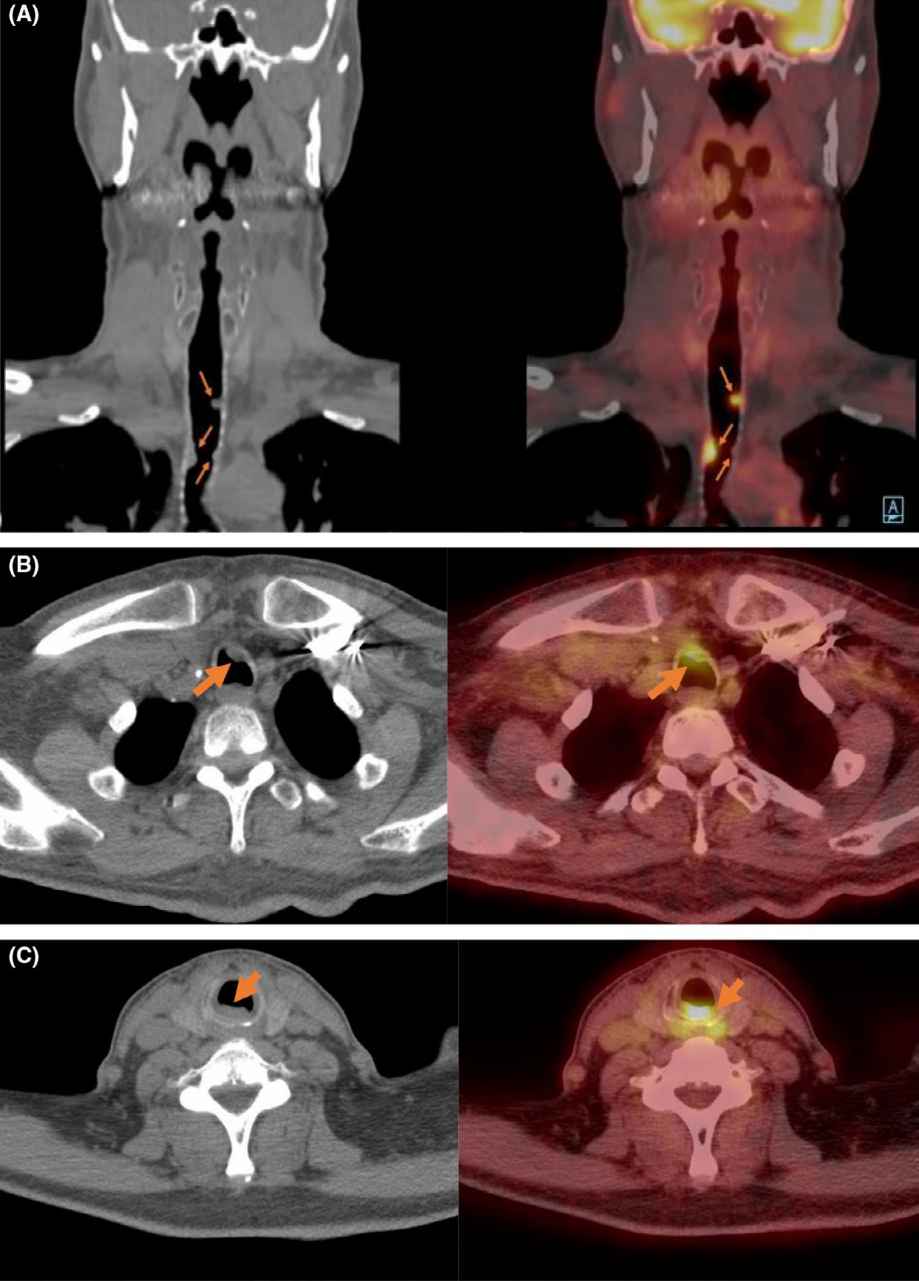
Radiographic development of intra‐luminal endobronchial metastases: A, interval development of multifocal FDG avid (SUV max 9.2) nodular soft masses on CT (left) and FDG‐PET (right) at the tracheal surface of patient 1. B, C, from Patient 2 showing CT and FDG‐PET lesions involving the subglottic larynx and ventral trachea
